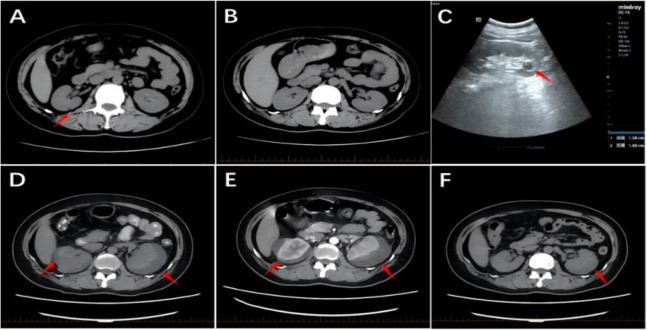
Abdominal imaging. (A) CT on March 3, 2022, shows a small cyst (approximately 0.4 cm, attenuation 2 HU) in the right kidney (red arrow). (B) CT on April 7, 2025, demonstrates no abnormalities in both kidneys. (C) Abdominal ultrasound on May 21, 2025, reveals a left renal cyst measuring approximately 1.38 cm × 1.40 cm (red arrow). (D) CT on July 7, 2025, indicates bilateral subcapsular hemorrhage and fluid collection, suggestive of hematoma (red arrow). (E) Contrast-enhanced CT on July 11, 2025, confirms bilateral subcapsular hemorrhage and fluid collection (red arrow). (F) CT on October 9, 2025, shows residual fluid collection in the left kidney (red arrow).
